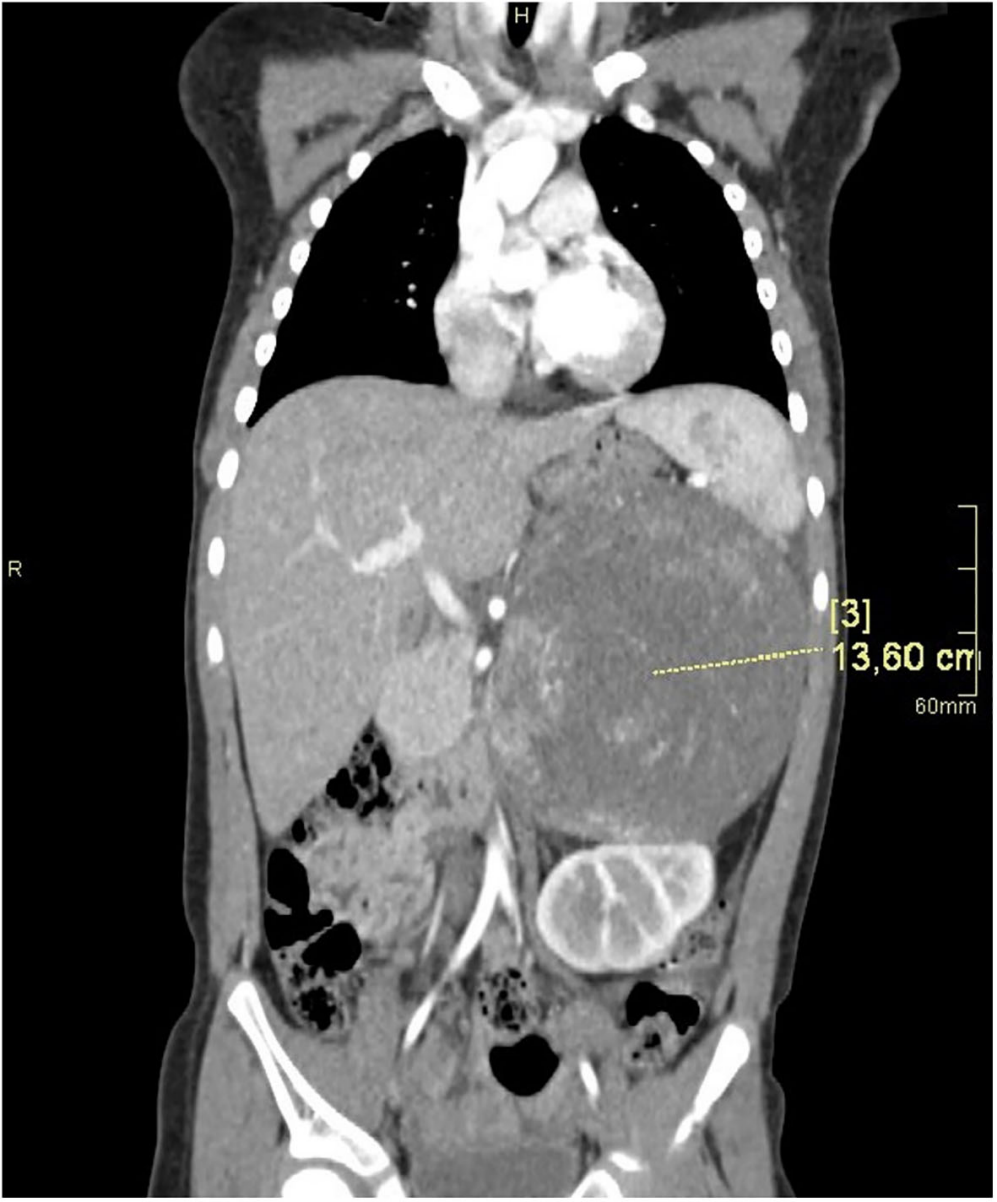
CT of the abdomen in the AP plane.
